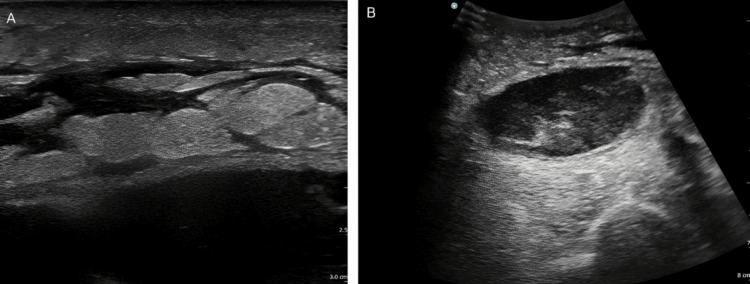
Superficial ultrasound image of the left thigh A high-frequency linear probe (3-12 MHz) was used for superficial ultrasonography, which revealed a cobblestone appearance in the subcutaneous tissue (A). Deep ultrasonography with a low-frequency convex probe (2-5 MHz) demonstrated a mixed hyperechoic and hypoechoic fluid collection along the deep fascial layer, suggestive of abscess formation (B).
